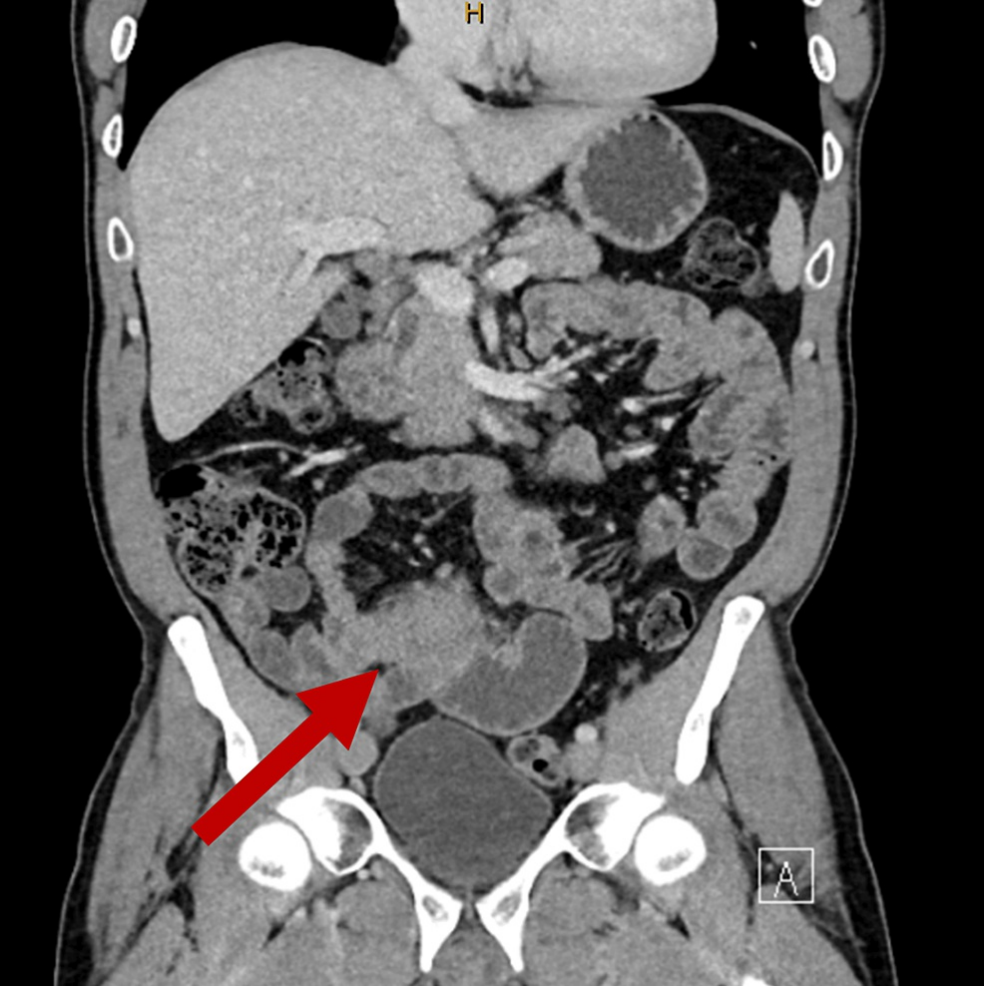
CT of the abdomen and pelvis with the red arrow demonstrating soft tissue mass extending into the mesentery with segmental thickening and high-grade stenosis of the mid and distal ileum, along with associated mesenteric lymphadenopathy and partial small bowel obstruction.
